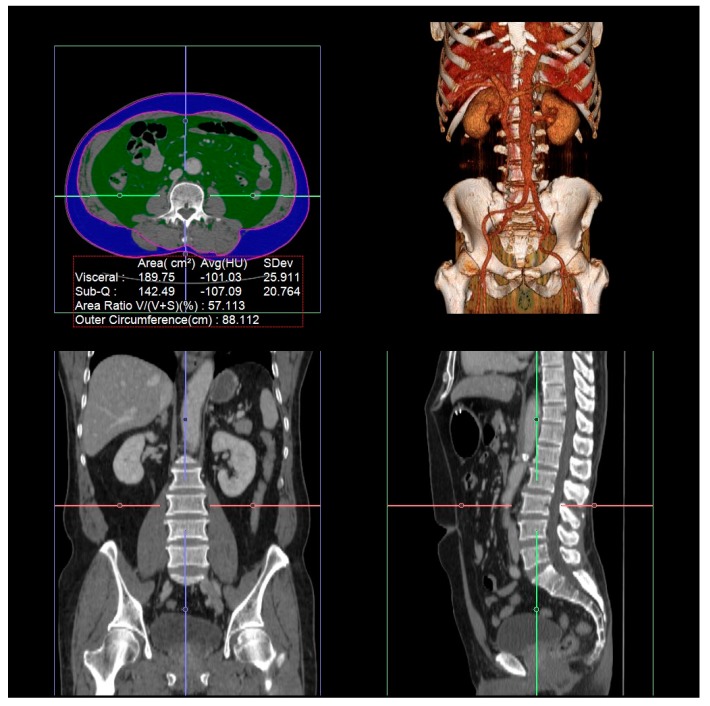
Example of body composition measurement at the level of the third lumbar vertebra on a computed tomography scan in the Hounsfield unit by AquariusNET Server.
